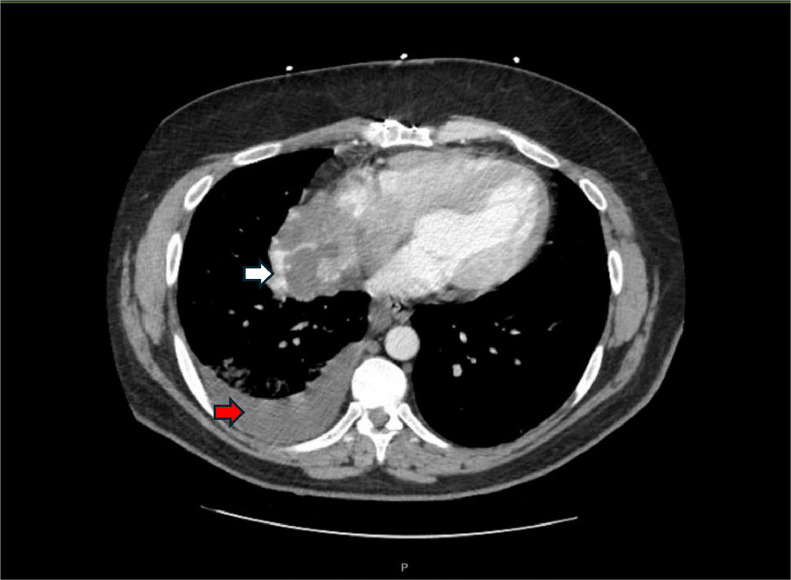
Axial contrast-enhanced CT chest showing a 6.6 × 6.3 cm irregular, heterogeneously enhancing, hypodense mass (white arrow) arising from the right atrial appendage. Moderate right pleural effusion is also noted (red arrow).
